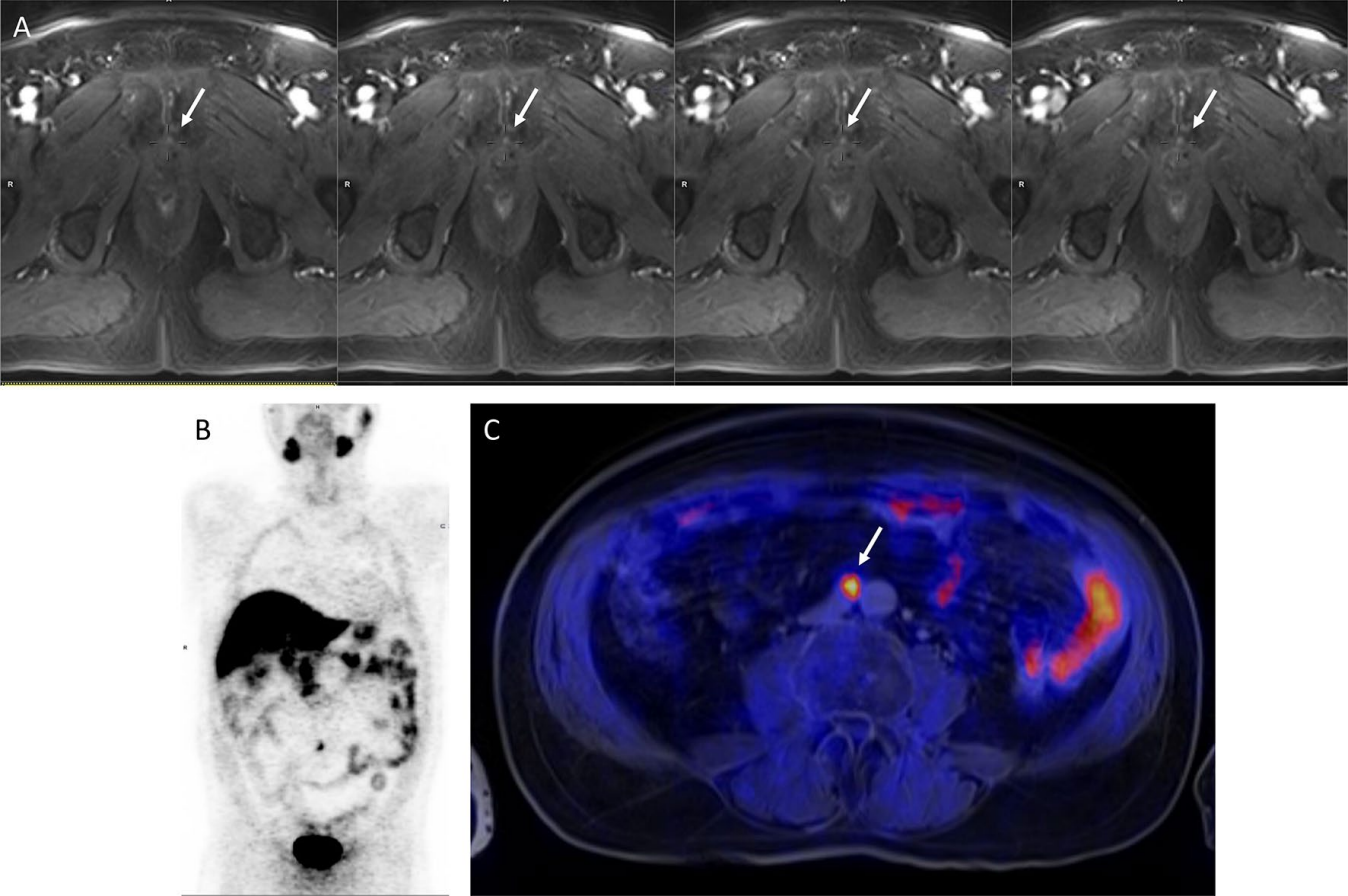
A 73-year-old man with a biochemical recurrence of prostate cancer (PSA = 2.46 ng/mL), treated with radical prostatectomy and pelvic lymph node dissection (pT3N0M0, Gleason Score: 9).a Magnetic resonance images showed the presence of an enhanced lesion in the prostatic fossae (arrow), compatible with a local recurrence. At PET (b, maximum intensity projection, MIP) and PET/MRI images (c), a focal uptake of FCH was found in a right lumbar lymph node (arrow), compatible with distant metastases
